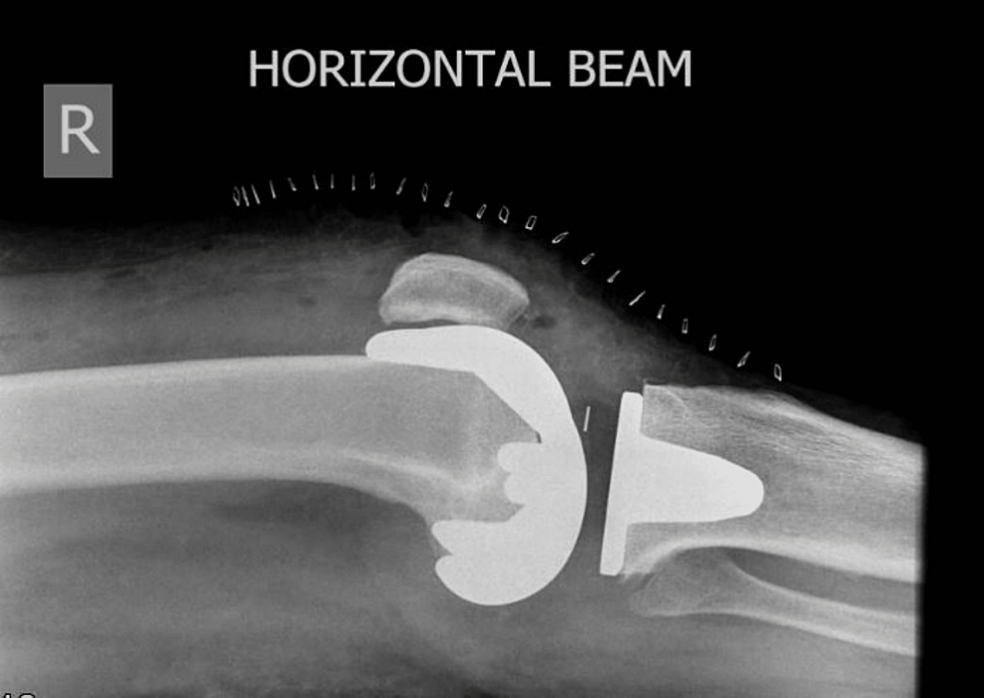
Postoperative X-ray, lateral view
